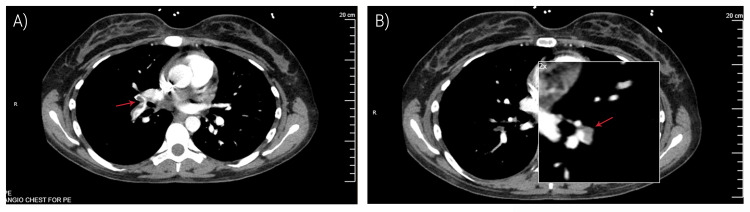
Bilateral pulmonary embolism on CT angiography (A) Filling defect within the interlobar artery on the right extending to the right middle and lower lobe segmental pulmonary arteries. (B) Subsegmental pulmonary arteries in the left lower lobe also demonstrated filling defects.
